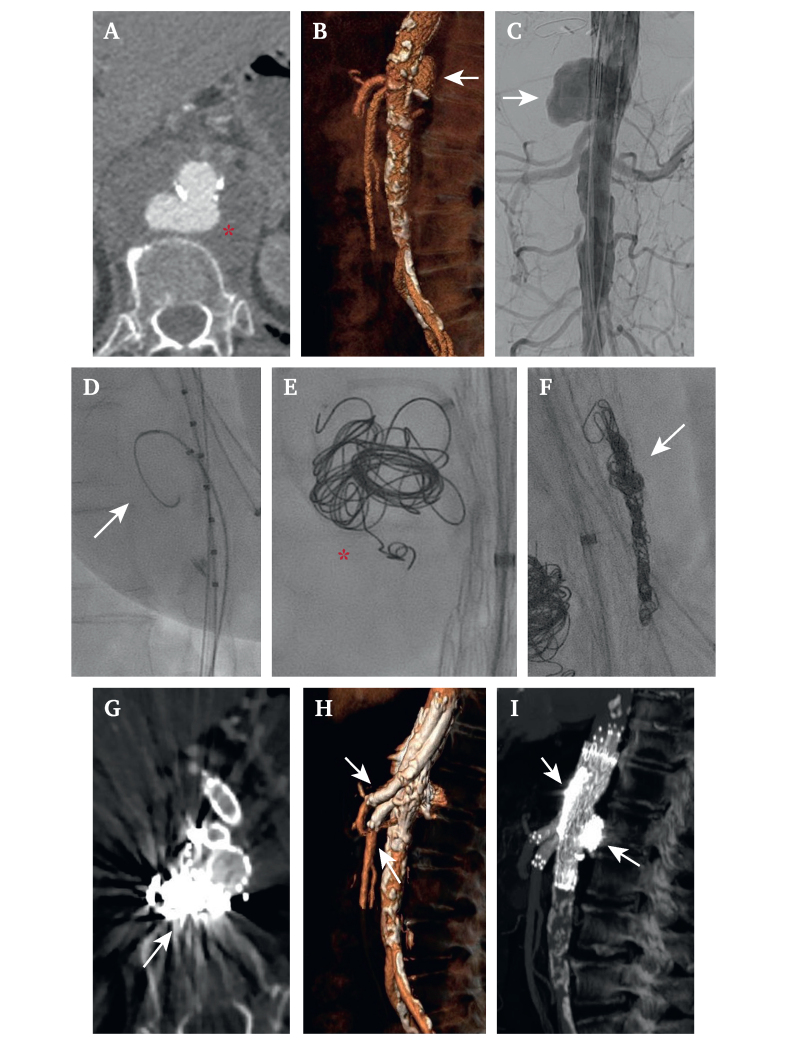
Procedure images. A. Anatomical characteristics of paravisceral aorta associated with symptomatic pseudoaneurysm with contained rupture of the posterior wall with peri-aortic haematoma. B. The pseudoaneurysm involved both zone 6 and zone 7, as shown in the pre-operative volume rendering reconstruction; and C. At the first intra-operative angiogram. D. Procedural step of the embolisation technique: the selected introducer sheath is parked into the pseudoaneurysm before introducing the main stent graft; E. after deployment of thoracic endograft coil embolisation of the pseudoaneurysm is performed; and F. Retrieval of the introducer sheath in the gutter channels and embolisation using detachable coils. G. Post-operative computed tomography angiography result with exclusion of the pseudoaneurysm in the axial projection. H. Details of the chimney revascularisation for the superior mesenteric and coeliac arteries (dialysis patient with planned sacrifice of both renal arteries), and I. Sagittal details of concomitant coil embolisation of the aortic pseudoaneurysm and the gutter channels.
